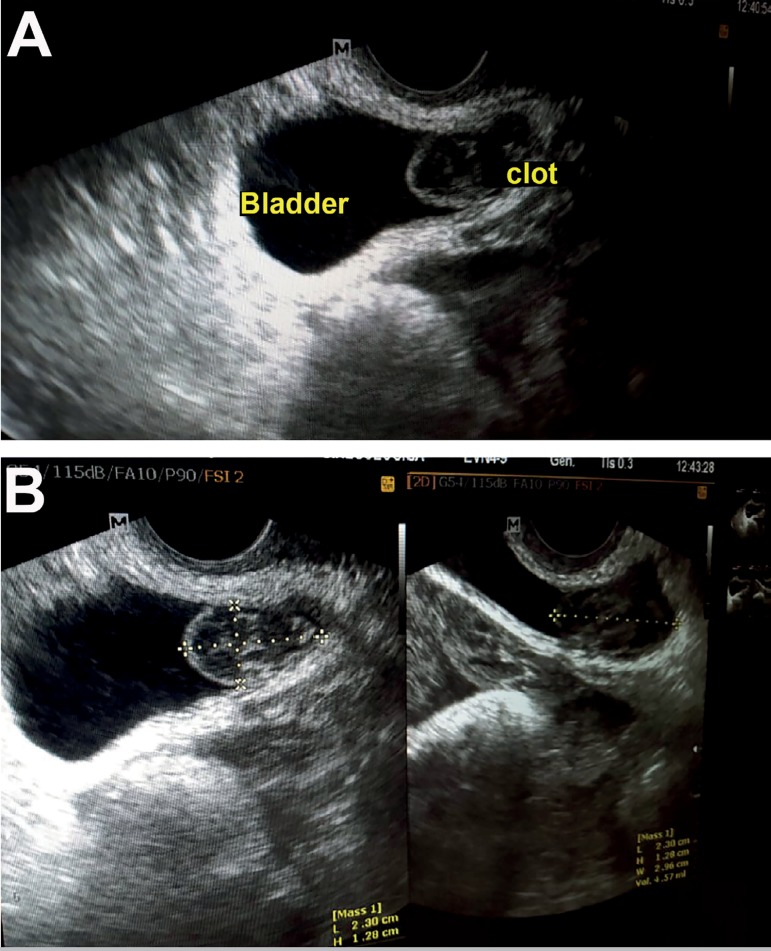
A and B. Transvaginal ultrasound examination performed one day after
